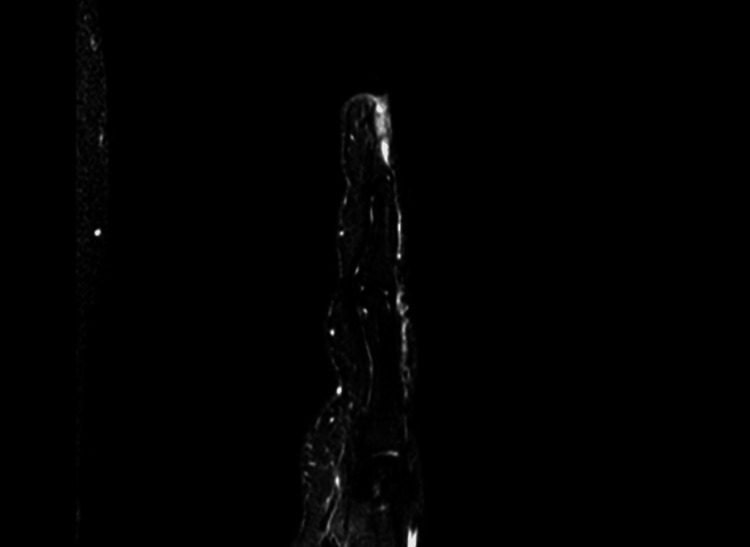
Sagittal MRI image showing the presence of a glomus tumor.
